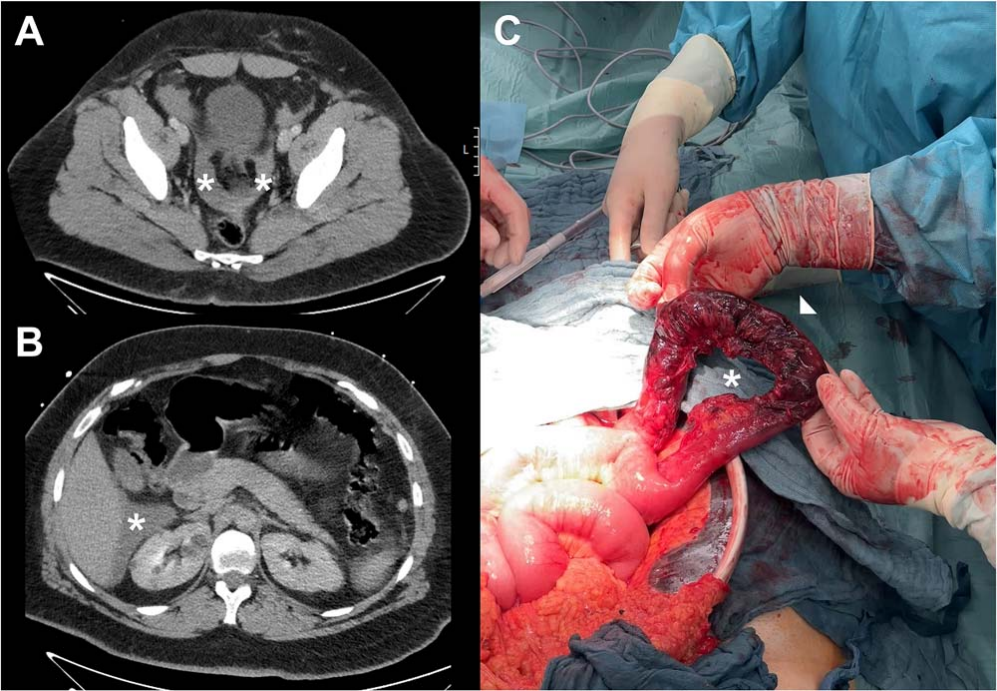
(A) Axial contrast-enhanced pelvic CT scan at patient’s admission and on (B) the second day post-trauma. Ascites is labeled by an asterisk. (C) Photograph (cranial-caudal orientation) of mesenteric injury (asterisk) with small bowel infarction (arrowhead).
